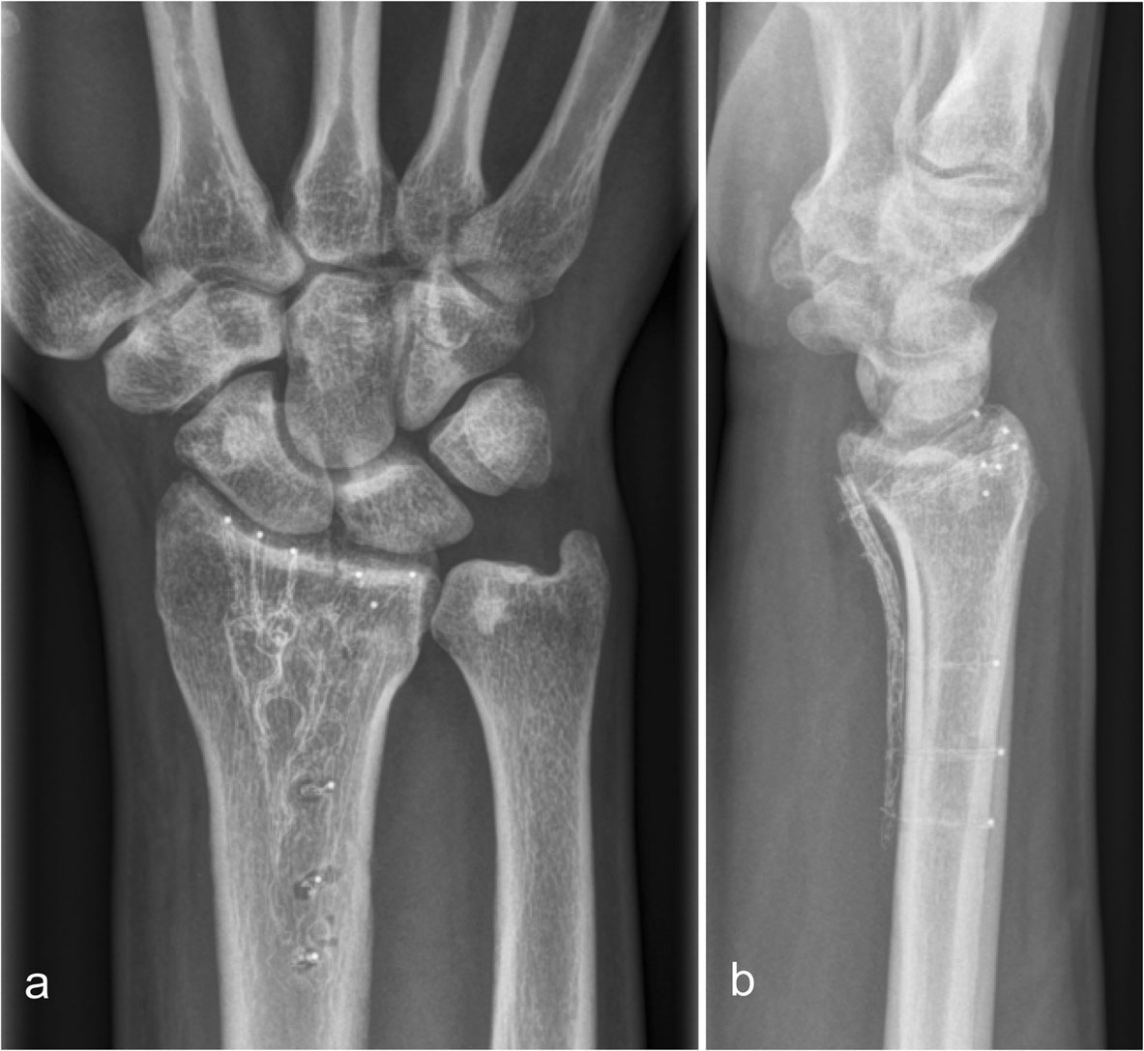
Distal radius fracture treated with a 2.7 mm Carbon/PEEK plate. X-ray 6 weeks after surgery anteroposterior (ap) (a) and lateral (lat) view (b)
